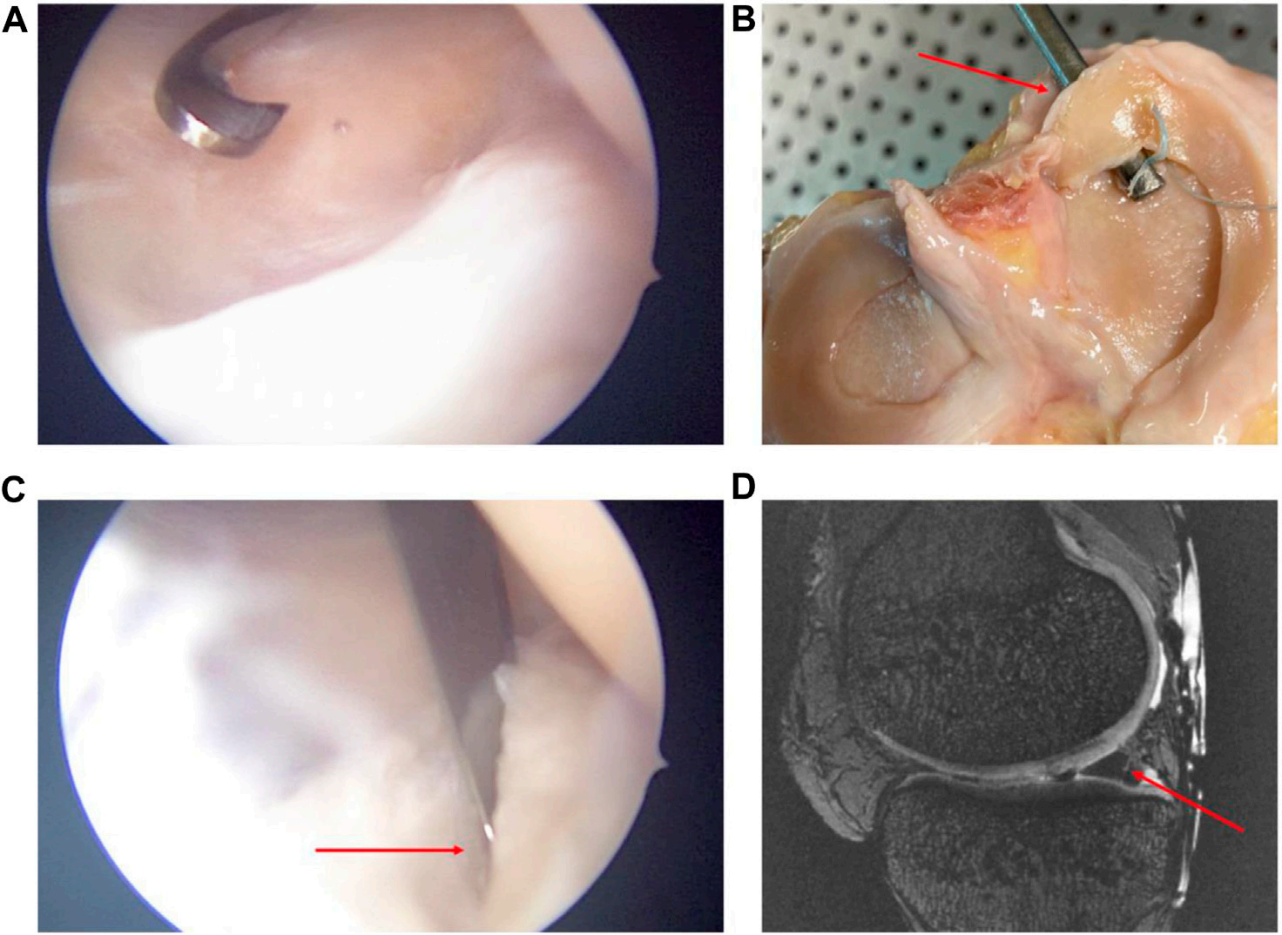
Arthroscopic views (A,B) of subtype 4 medial meniscus RL creation by a posteromedial approach. Open view of the knee and its meniscal RL injury after experimentation (C). MRI sagittal view in sequence T2 DESS of the subtype 4 RL injury (D). Red arrows show the subtype 4 RL.
